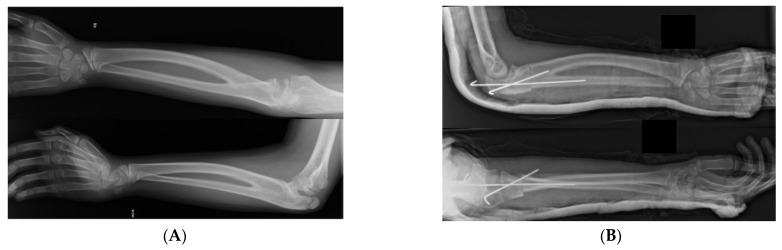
(A) Bilateral congenital radioulnar synostosis. Preoperative X-ray. (B) Osteotomy through the synostosis going simple and easy to fix with 2 KW. Postoperative X-ray.
